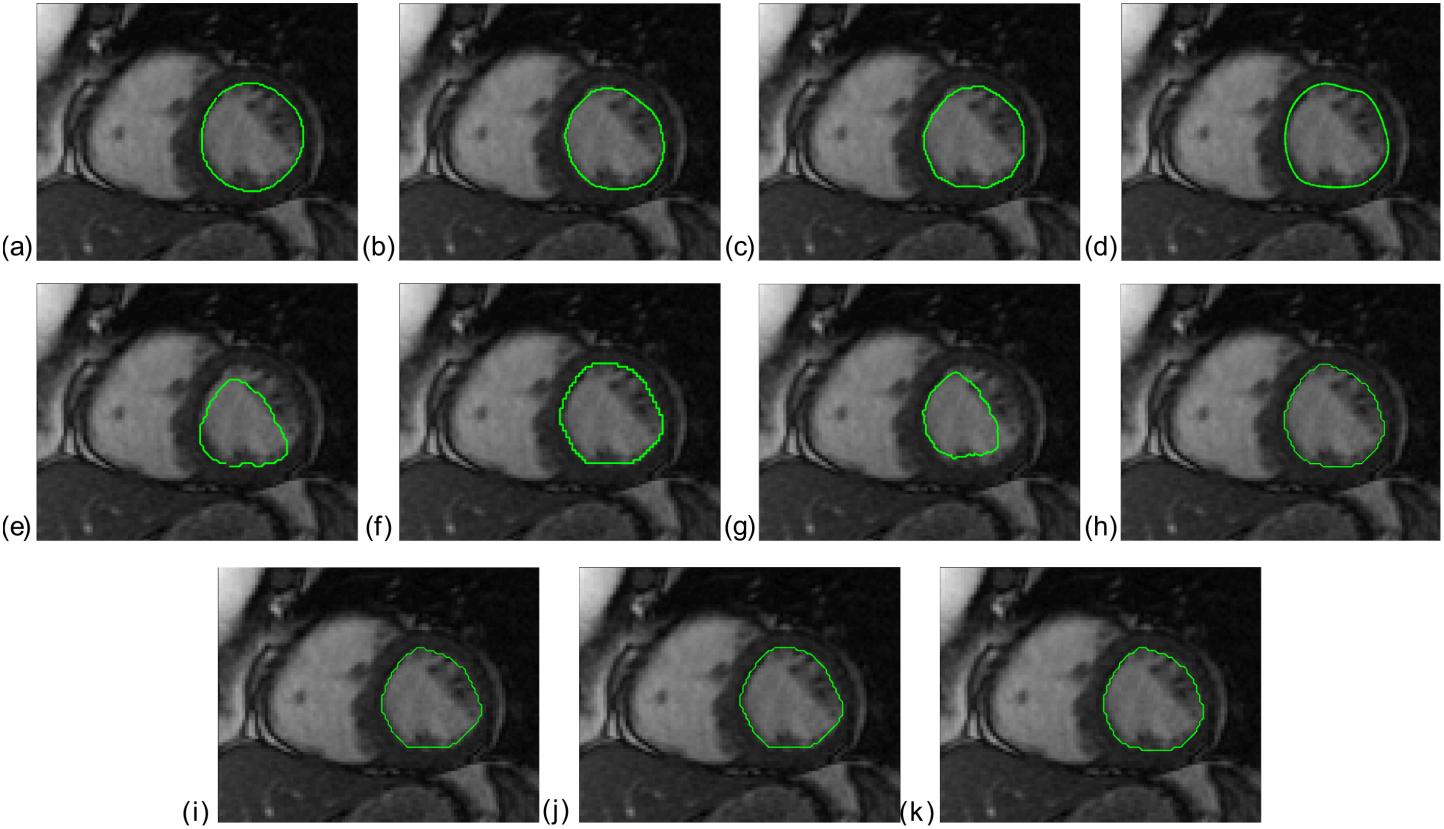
Median cine MRI slice at end-diastole with superimposed contours of the LV (green line). *M*1 to *M*8 are represented from (a) to (h) and three different combinations of the STAPLE algorithm, *MS*45678, *MS*456 and *MS*4578 are represented from (i) to (k).
